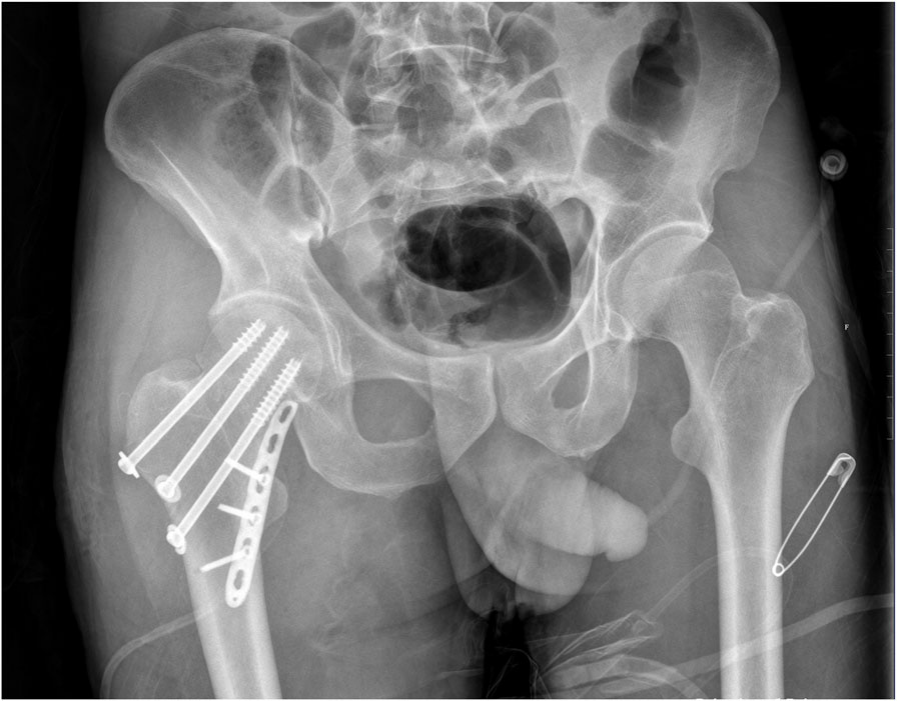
Representative X-ray image of one clinical case who was treated for vertical femoral neck fracture using three cannulated screws and a buttress plate without a proximal screw
